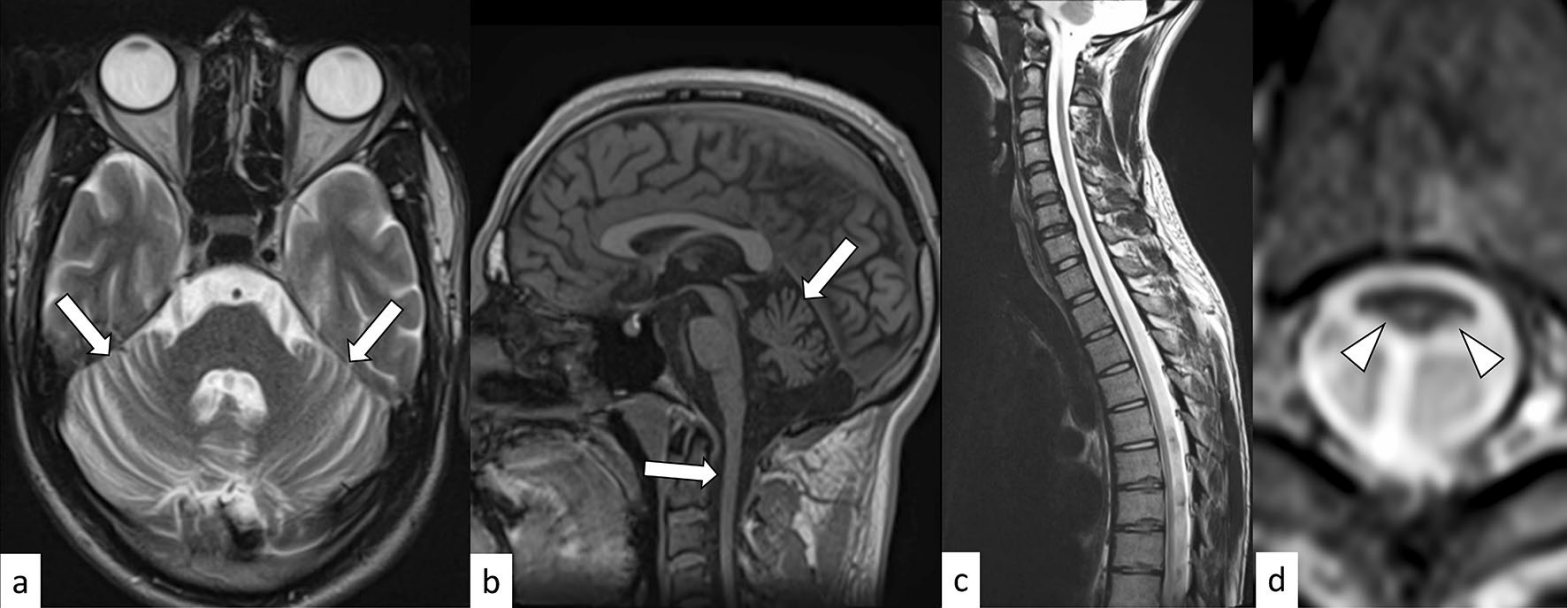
A 22-year-old man with Friedreich’s ataxia. T2-weighted axial image a and T1-weighted sagittal image b show atrophy of the cerebellum and brainstem (arrows). T2-weighted sagittal image shows diffuse spinal atrophy (c). T2-weighted axial image shows hyperintensity in the bilateral dorsolateral funiculi (d, arrowheads)
